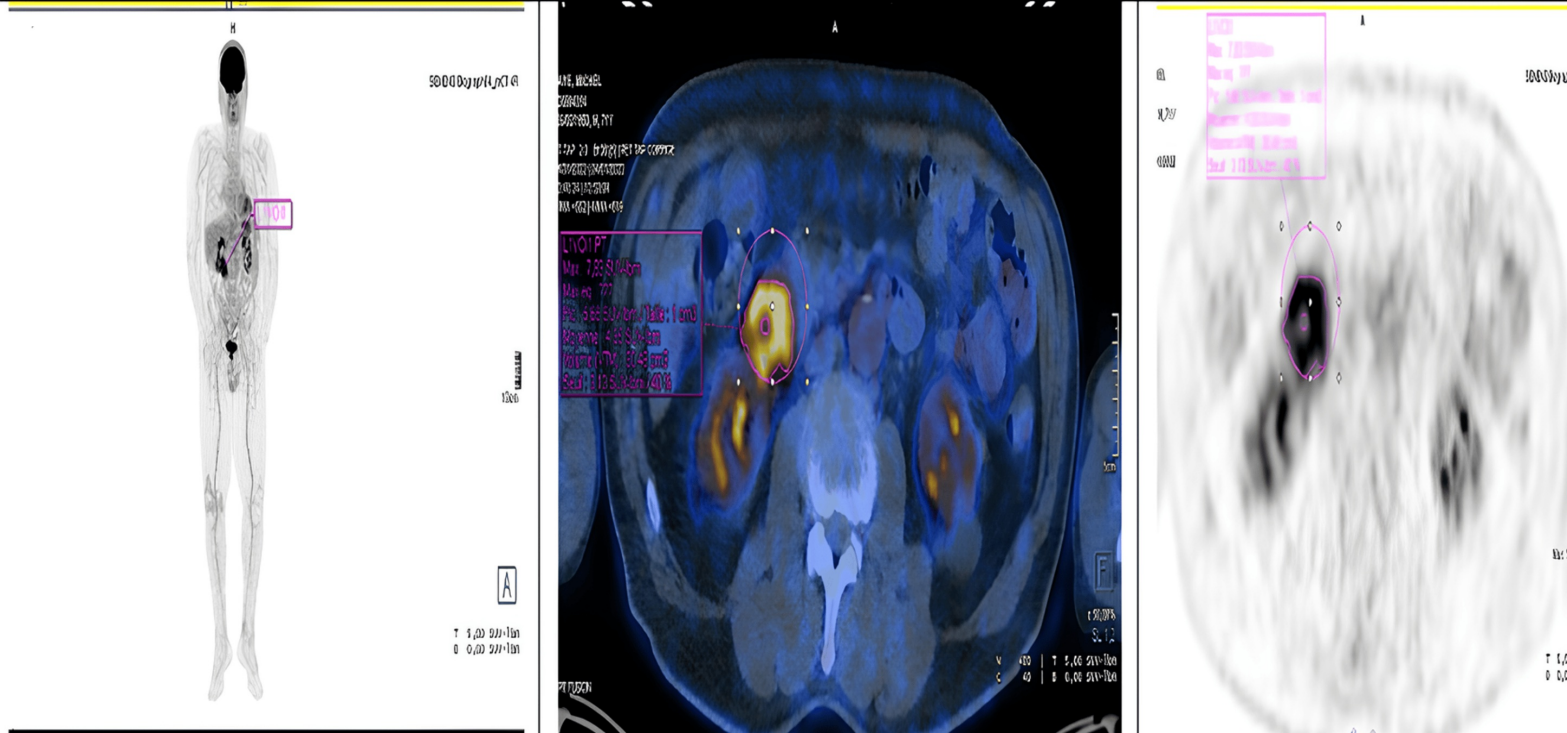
PET/CT at diagnosis showed pathological hypermetabolism at the height of the tumoral infiltration of the second duodenum (SUVmax: 16.8) PET/CT, positron emission tomography/computed tomography; SUVmax, maximum standardized uptake value
